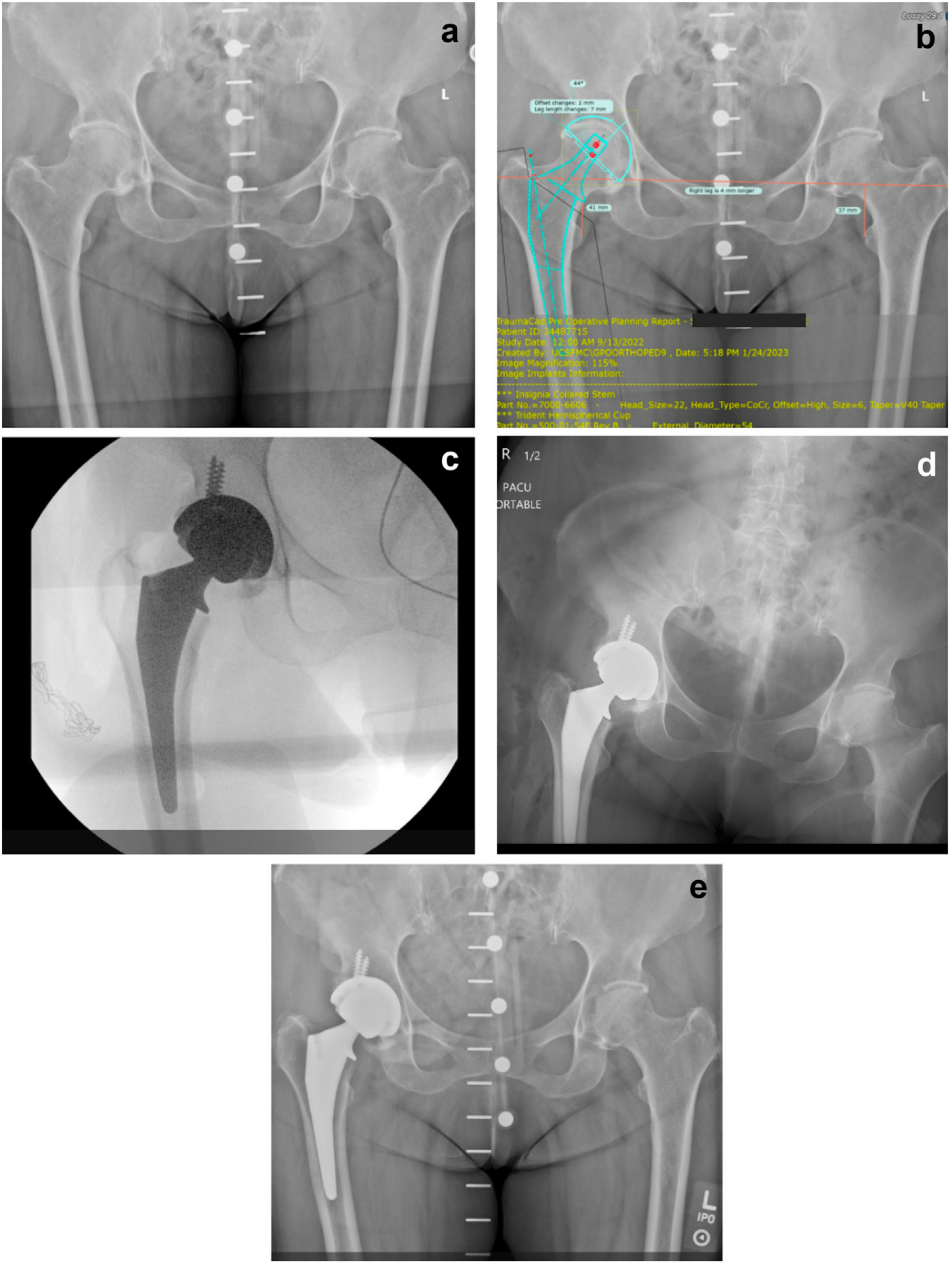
Case with 2 sizes under the template with a collared triple taper stem in a 75-year-old female. (a) Preoperative radiograph; (b) Preoperative template; (c) Intraoperative fluoroscopy; (d) Immediate postoperative radiograph; (e) 6 weeks postoperative radiograph.
